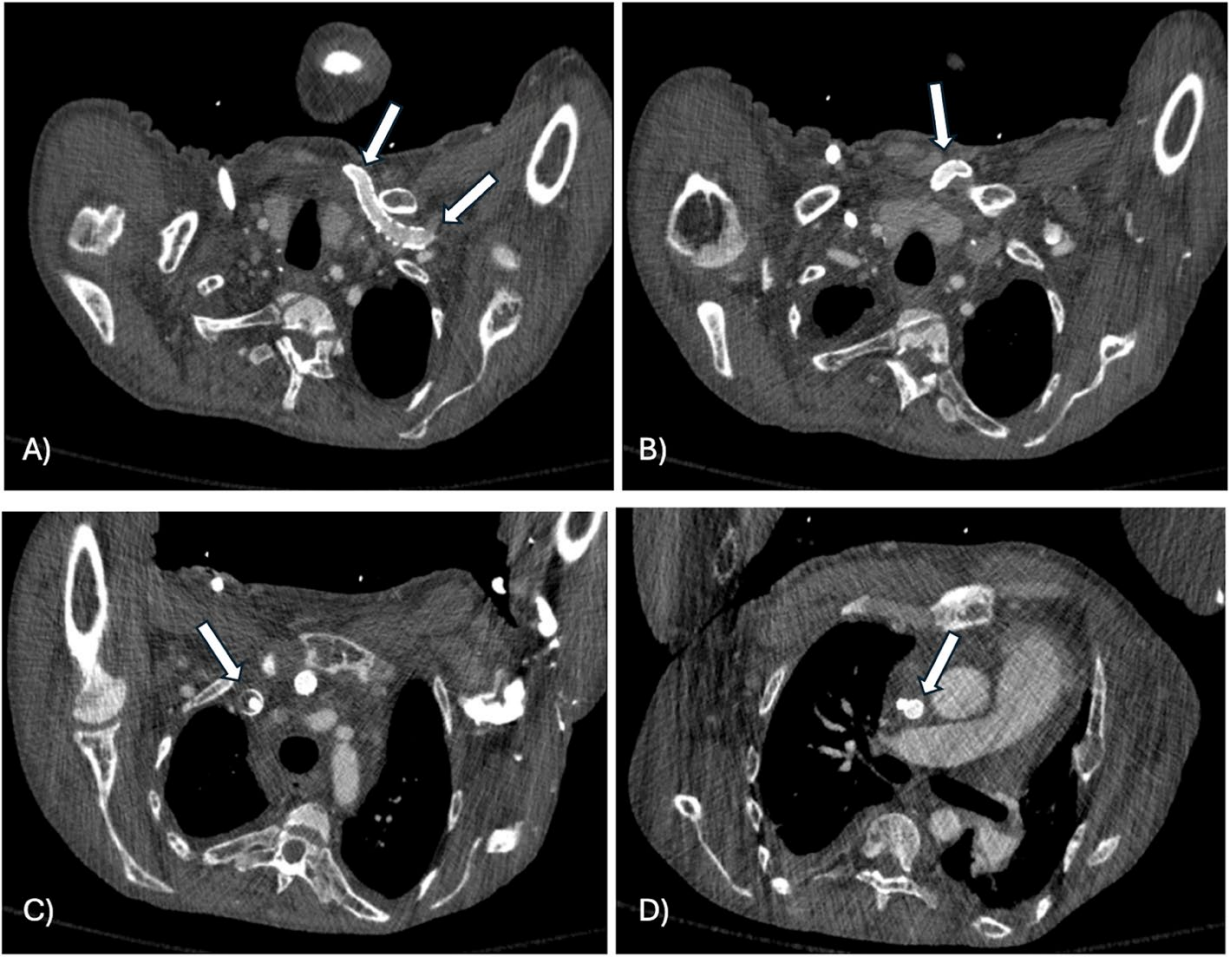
Serial axial contrast enhanced CT images of the chest from cranial (A) through caudal (D) show patency of the left subclavian through SVC stents (white arrows) at 3 months without significant filling defect
